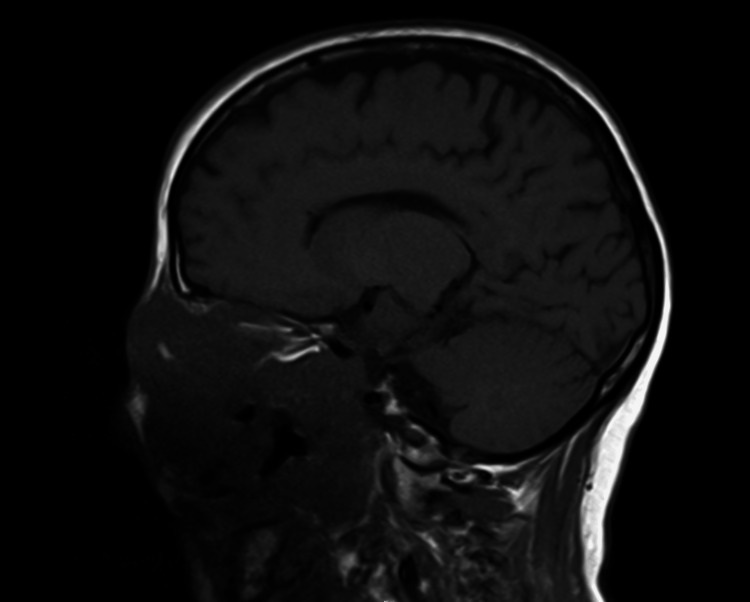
MRI brain with contrast MRI brain, sagittal plane, demonstrating the epidural expansion of the neoplasm along the floor of the anterior cranial fossa (left>right) with the abutment of the bilateral inferior frontal lobes. This view also allows for visualization of the epidural expansion into the left middle cranial fossa inseparable from the underlying anterior left frontal lobe.
